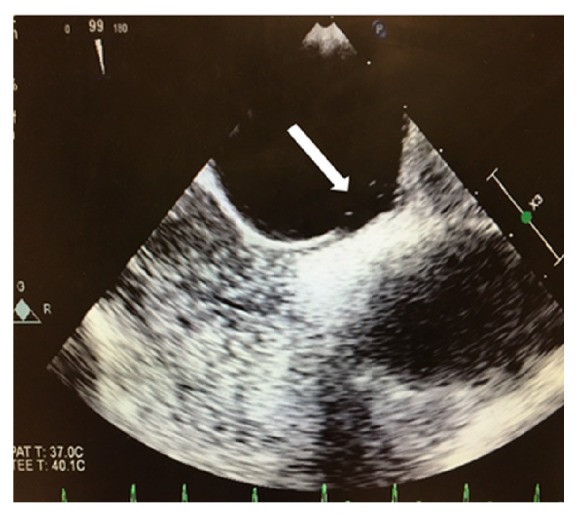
White arrow showing 3 bubbles crossing the atrial septum on transesophageal echocardiogram.
